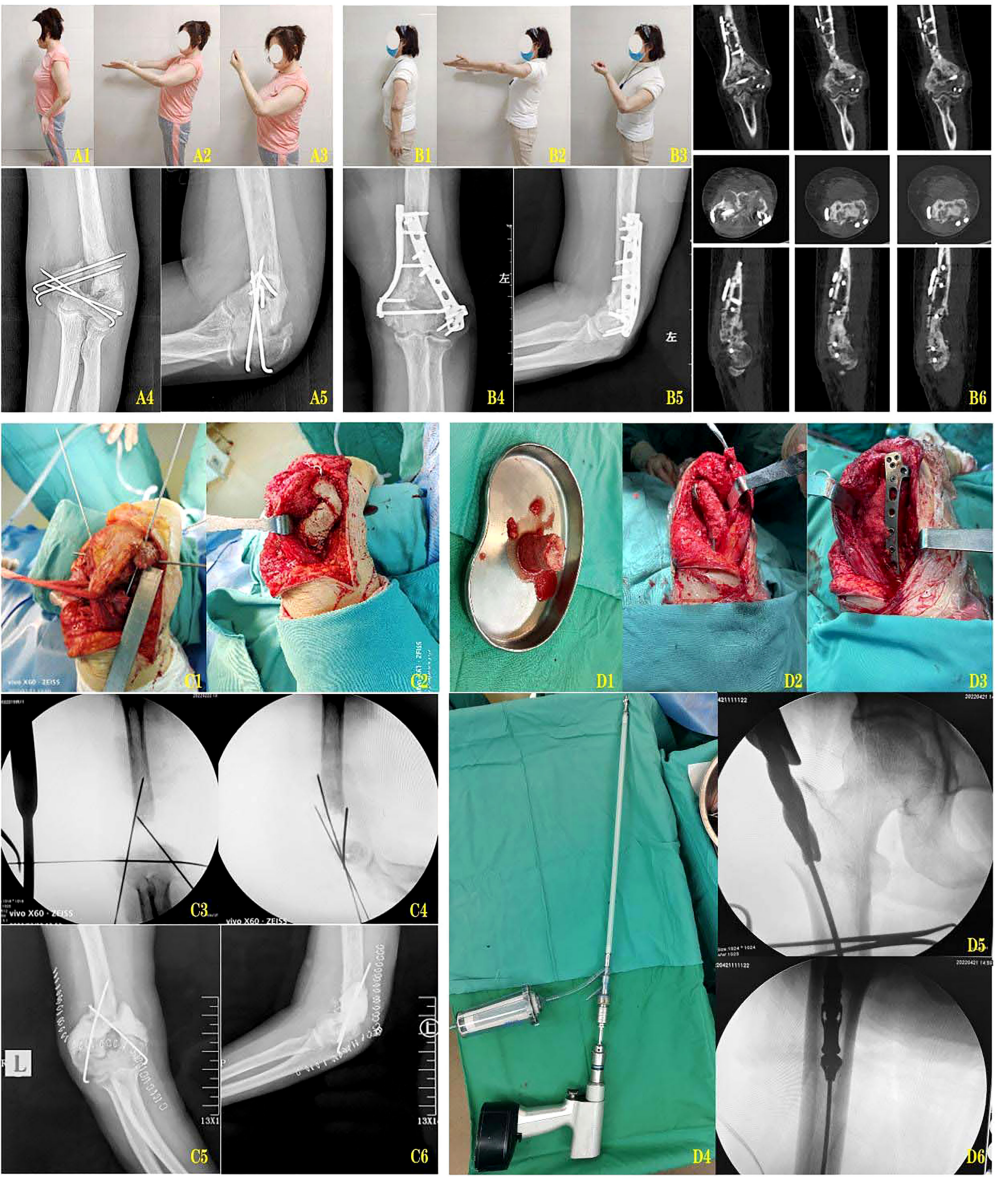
(A1-A3) The posterior function of the fixation of the fracture. (A4-A5) The left elbow of the posterior left elbow and the lateral X-ray. (B1-B3) 10 months after Masquelet induction and RIA bone graft reconstruction. (B4, B5) Posterior lateral X-rays of the left elbow joint at 10 months after internal fixation reconstruction. (B6) CT scan at 10 months after internal fixation reconstruction. (C1, C2) The membrane induced phase of the process. (C3, C4) The image of the membrane induced phase. (C5, C6) The membrane is induced by the left elbow and the lateral position X-ray. (D1) The pine bone clay removed from the myotor. (D2, D3) Use the medullary bone graft bone to rebuild the distal humerus. (D4) The use of the RIA reaming device is widely used. (D5, D6) RIA was expanded with the image of the bone.
